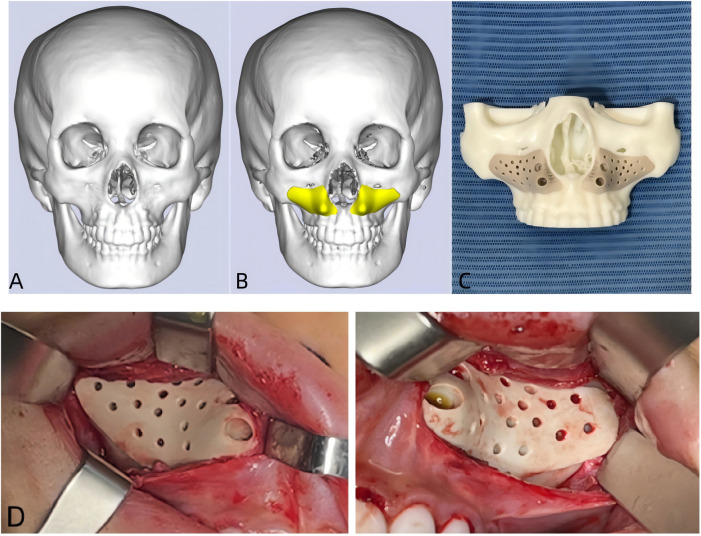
Representative case 1: preoperative defect analysis, virtual design, manufactured implant, and intraoperative fit. (A) CT showing PARA-I, INFRA-I, and MALAR deficiency. (B) Virtual implant design based on patient goals. (C) Final CAD/CAM-manufactured IPPSI. (D) Intraoperative placement.
